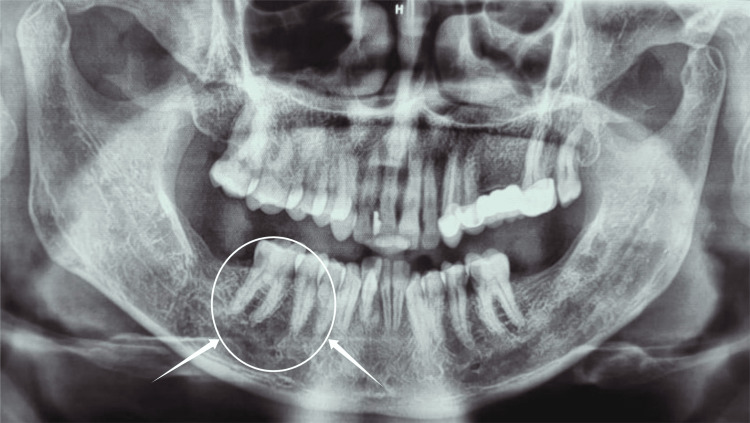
Orthopantomogram image: arrows showing no bone involvement in the regions of 45 and 46
